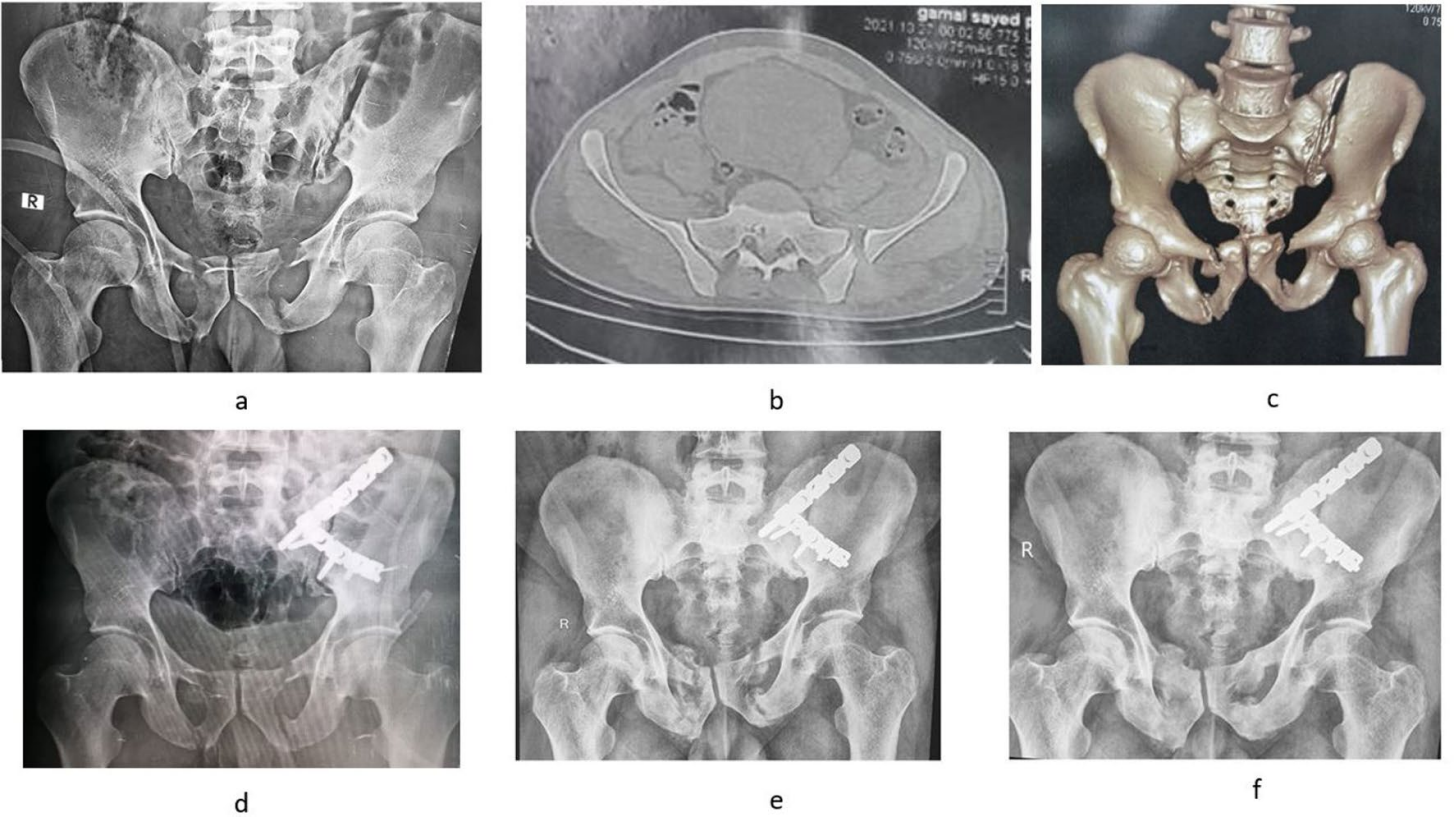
A LC 2 case (Day 1) with tetra-ramic fracture. Posterior-only fixation was done. Preoperative AP X-ray (a), axial CT (b) and 3D reconstruction (c). Immediate post-operative AP X-ray is shown (d). Fracture showed maximum secondary displacement of 5.8 mm in AP view on eight-week follow-up (e). The fracture healed with no further displacement; follow-up AP view at one year (f)
